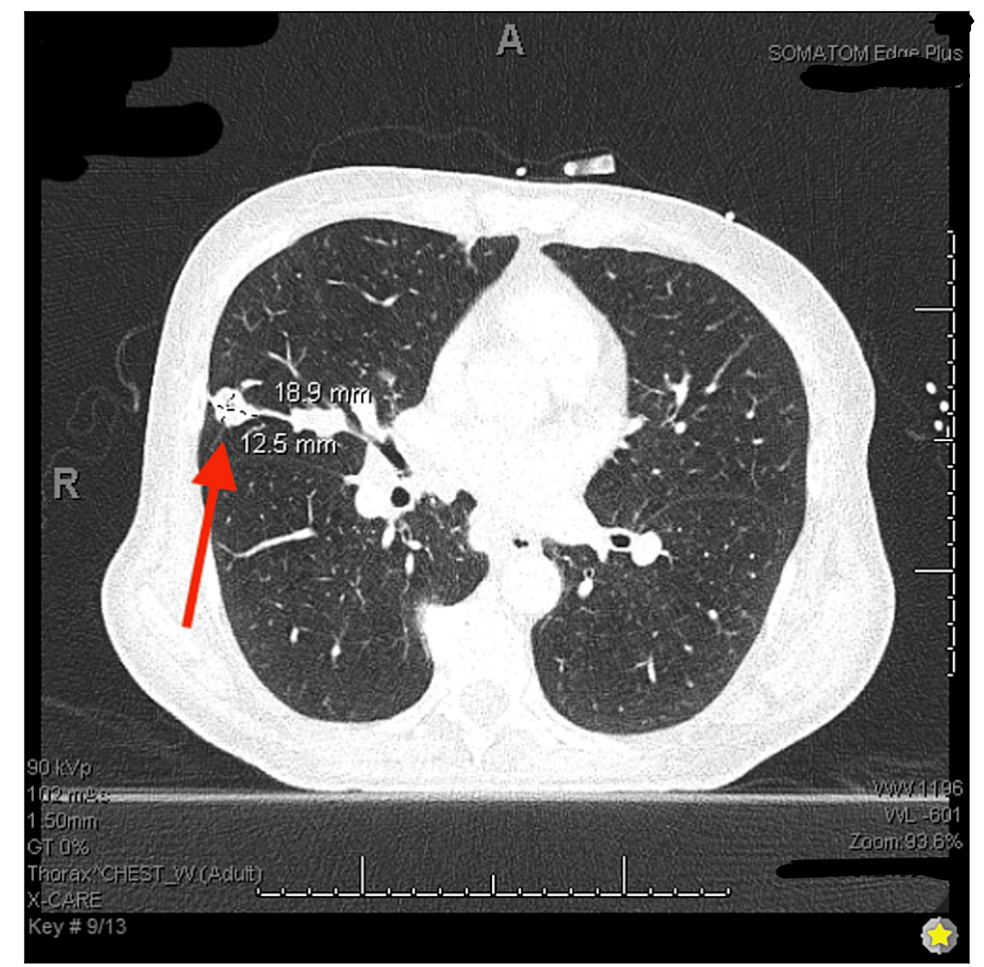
CT chest with iodine contrast showing subpleural right middle lobe lung cavitary nodule (1.3 cm x 1.9 cm lung mass).
